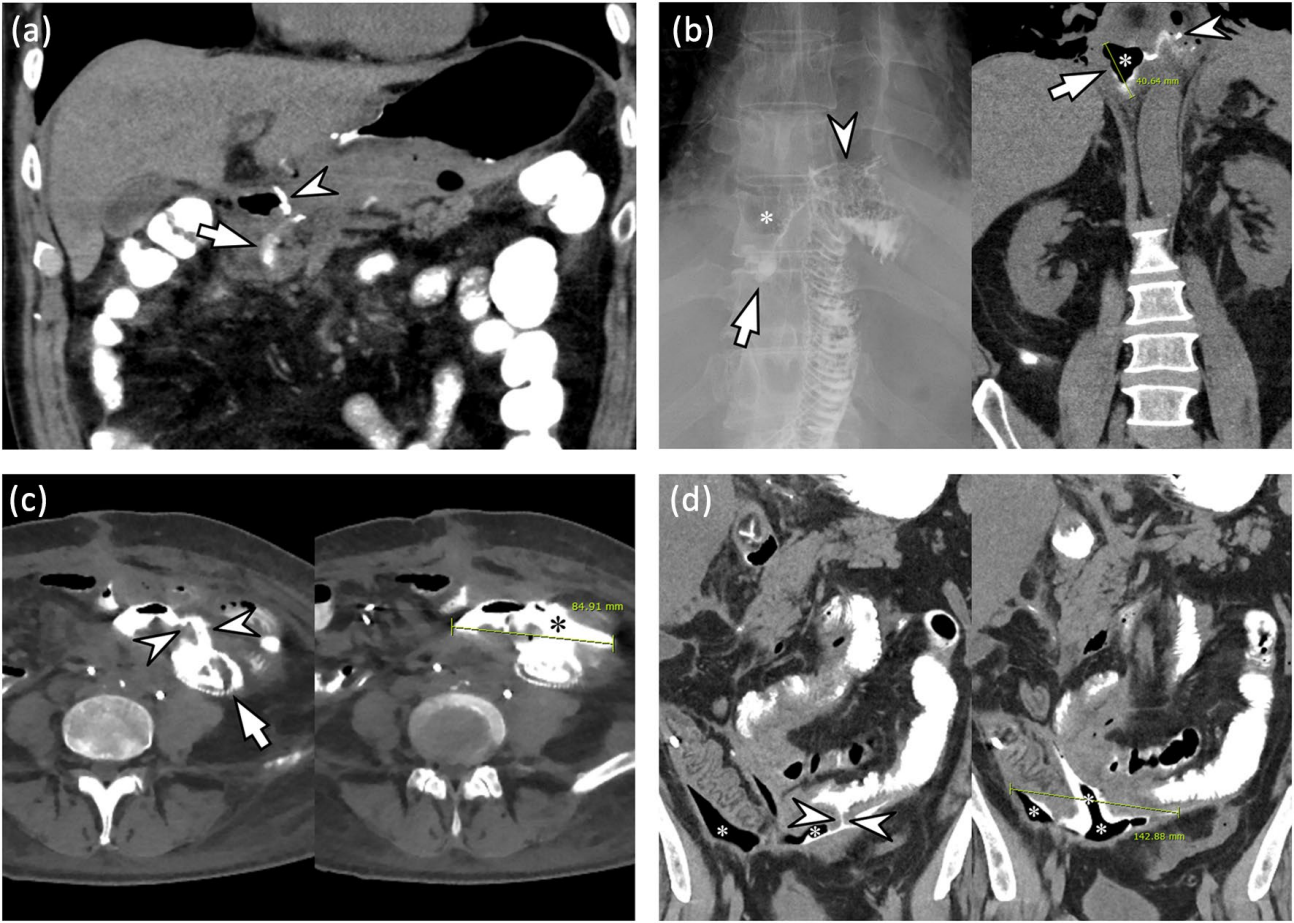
Representative examples for grading gastrointestinal leakage after gastrointestinal surgery. (a) Grade 1: minimal (pinpoint or scanty < 2 cm fluid). The patient underwent distal gastrectomy with Billroth I anastomosis for stomach cancer. On a coronal non-contrast CT image obtained immediately after fluoroscopic examination, there is a scanty (< 2 cm) extraluminal contrast collection (arrow) at the inferior aspect of gastroduodenostomy (arrowhead). (b) Grade 2: small (single and 2–5 cm fluid collection). The patient underwent total gastrectomy with esophagojejunostomy for stomach cancer. The fluoroscopic image (left) and coronal non-contrast CT image (right) obtained after fluoroscopic examination reveals contrast leakage (arrow) at the right side of the esophagojejunostomy site (arrowhead). Note a 4 cm small air-containing cavity (*) with small contrast material around the leakage site. (c) Grade 3: intermediate (5–10 cm fluid collection). The patient underwent metallic stent insertion for sigmoid colon obstruction due to sclerosing mesenteritis. On axial non-contrast CT images obtained immediately after fluoroscopic examination, there is a contrast leakage (arrowheads) from proximal end of metallic stent (arrow) at sigmoid colon. Note an 8.5 cm fluid cavity (*) filled with extravasated contrast material around the leakage site. (d) Grade 4: large (> 10 cm or disseminated fluid collection). The patient underwent right hemicolectomy for ascending colon cancer. On coronal non-contrast CT images obtained immediately after fluoroscopic examination, there is a small defect at ileum (arrowheads). Note a > 14 cm large air and fluid filled cavity containing extravasated contrast material (*) around the leakage site.
